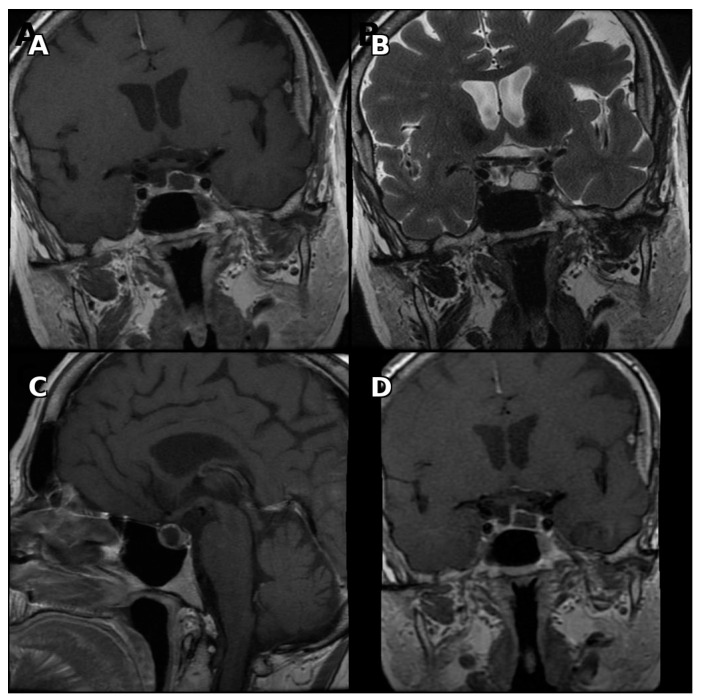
Pituitary MRI of a patient with cystic pituitary adenoma. (A) Contrast-enhanced coronal T1-weighted image demonstrating paramedian localization. (B) Coronal T2-weighted image demonstrating a hypointense rim surrounding the cystic lesion. (C) Contrast-enhanced sagittal T1-weighted image demonstrating anterior localization. (D) Delayed contrast-enhanced coronal T1-weighted image demonstrating lack of internal enhancement.
